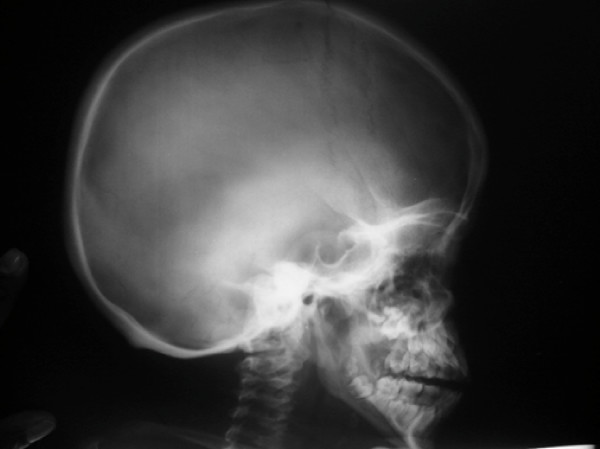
Anteroposterior and lateral X-ray of the skull showing a 'J' shaped sella turcica.
